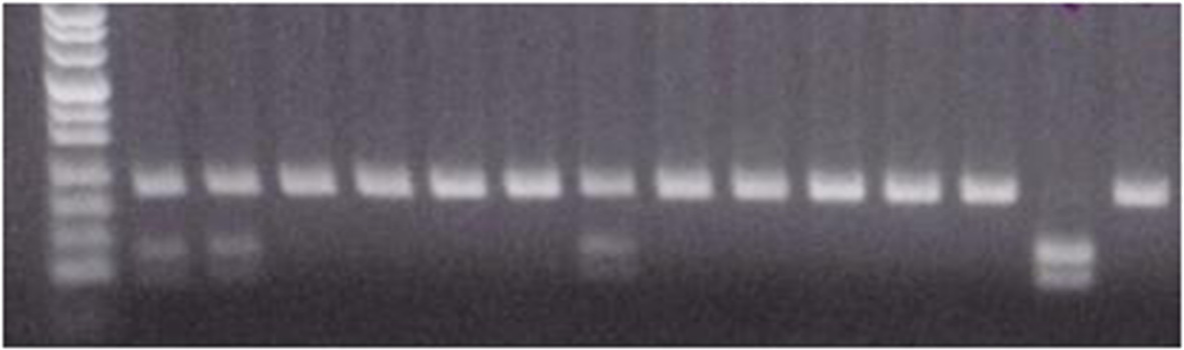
Identification of rs5742909 polymorphism of CTLA4 gene. Lanes: M – DNA length marker (pUC Mix Marker 8, MBI Fermentas), 1, 2, 7 – CT heterozygotes, 3, 4, 5, 6, 8, 9, 10, 11, 12, 14 – CC homozygotes, 13 – TT homozygote.
